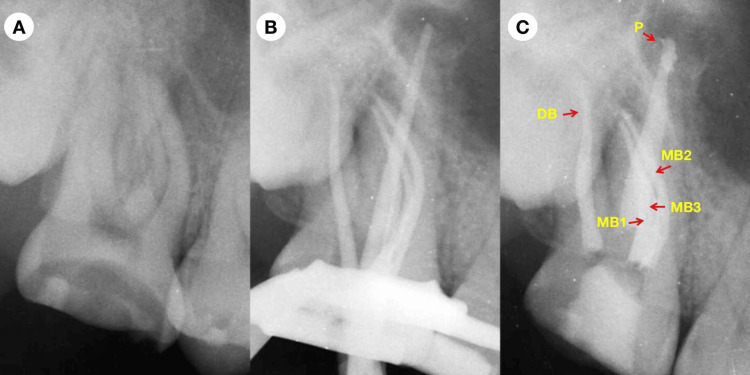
Pre-operative (A), cone-fit (B), and post-obturation (C) radiographs. MB1: mesial buccal first canal; MB2: mesial buccal second canal; MB3: mesial buccal third canal; DB: distal buccal canal; P: palatal canal
